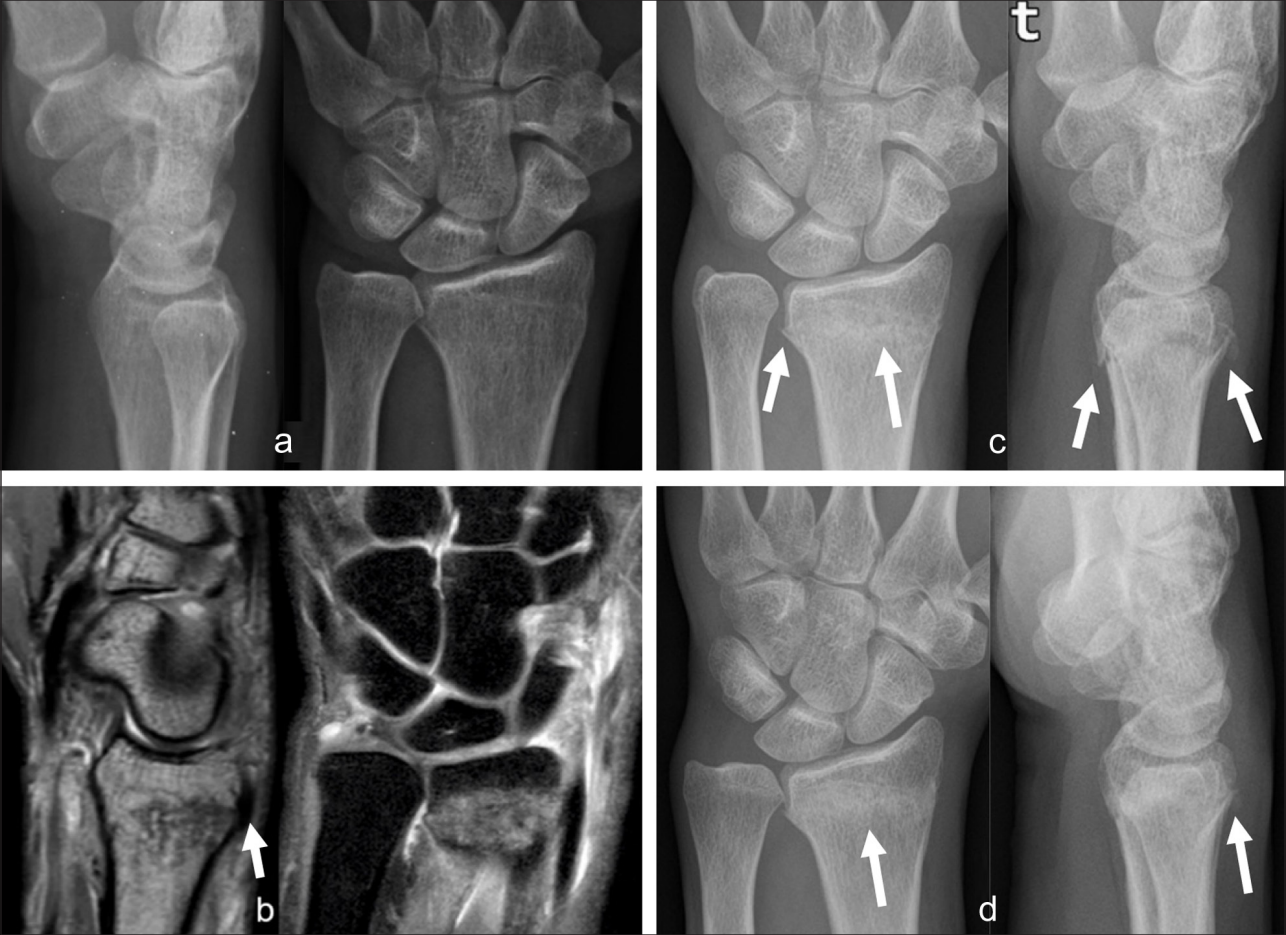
Occult radius fracture detected by MRI. The radiographs, (a) lateral and anteroposterior views, obtained the day after trauma were negative. Three days later, sagittal PD and coronal PDFS, (b) MR images revealed a nondisplaced radius fracture with extensive bone marrow edema and posterior cortical irregularity on the sagittal view (arrow). Control radiographs (AP and lateral views) obtained one month (c, arrows) and two months (d, arrows) post trauma depict consolidation signs, better seen on d (arrow).
